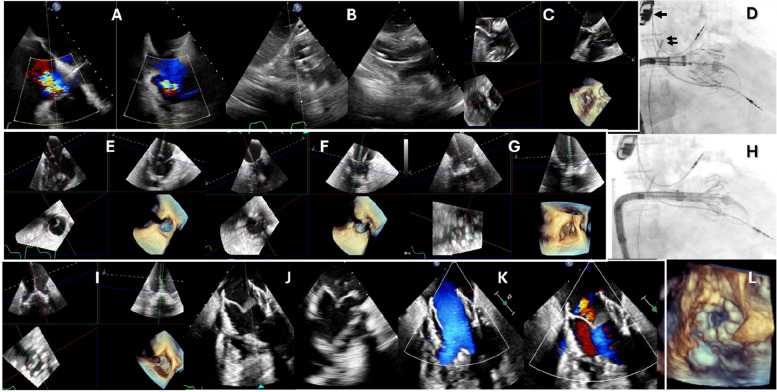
Case of transcatheter tricuspid valve replacement with the EVOQUE system (Edwards Lifesciences), for cardiac implantable electronic device–related severe tricuspid regurgitation (TR). A The mid-esophageal biplane views of the tricuspid valve (TV) with significant TR are shown. B The biplane transgastric transesophageal echocardiography (TEE) views of the TV are suboptimal to visualize the stiff wire in the right ventricle (RV); hence, C 3D intracardiac echocardiography (ICE; Veri Sight Pro, Philips) is used to guide the procedure. D The locations of the ICE catheter in the right atrium (RA; double black arrow) and the TEE probe (single black arrow) are shown in the fluoroscopic image. E–G The 3D multiplanar reconstruction (MPR)-derived from the 3D ICE images are shown with the valve delivery system entering deep in E the RV, F mid RV, and G close to the TV, this allows fine adjustment of device depth, position and alignment. I The nine anchors of the valve are exposed, and the engagement of the leaflets are shown in the coronal plane of the 3D MPR. (H) The fluoroscopic appearance of this step is shown. J Full ventricular expansion is followed by atrial expansion and the valve is deployed under ICE guidance (biplane ICE image of the fully deployed valve is shown). (K) ICE color flow Doppler shows unobstructed diastolic TV flow and mild transvalvular TR. (L) The *en face* view of deployed valve from RA perspective under 3D TEE
